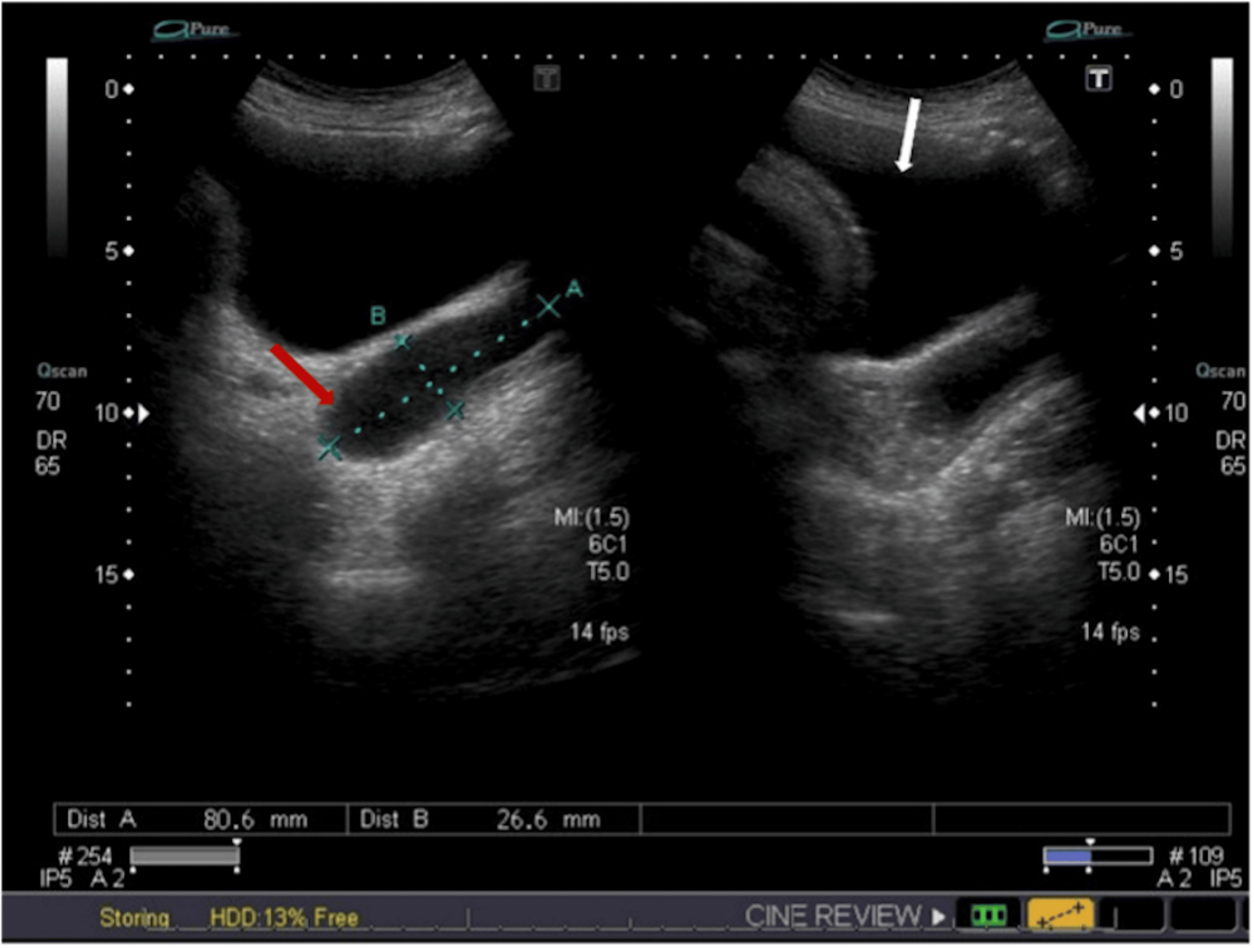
Targeted pelvic ultrasonography showing a cyst (red arrow) located in the upper vagina and extending to the cervix, with a measurement of approximately 8 cm in length, indicated by the blue line (A). The white arrow points to the urinary bladder.
